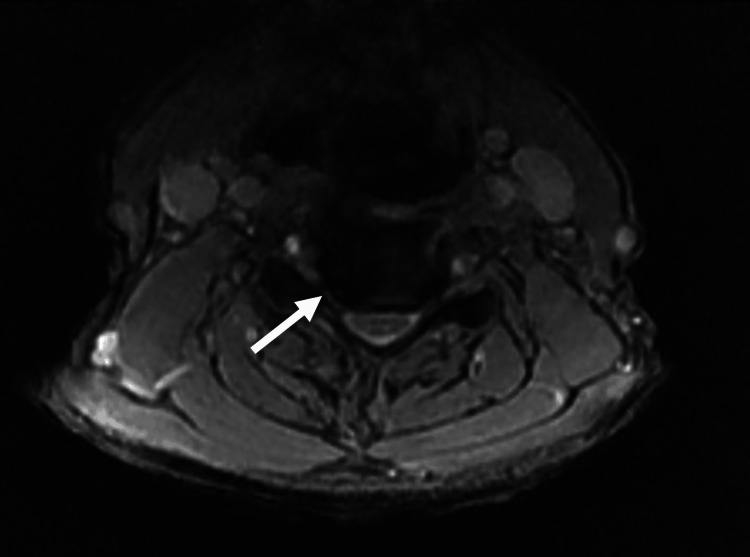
An axial T2W image at the level of C4-C5 showing a right-sided neuroforaminal stenosis (arrow) T2W: T2-weighted
